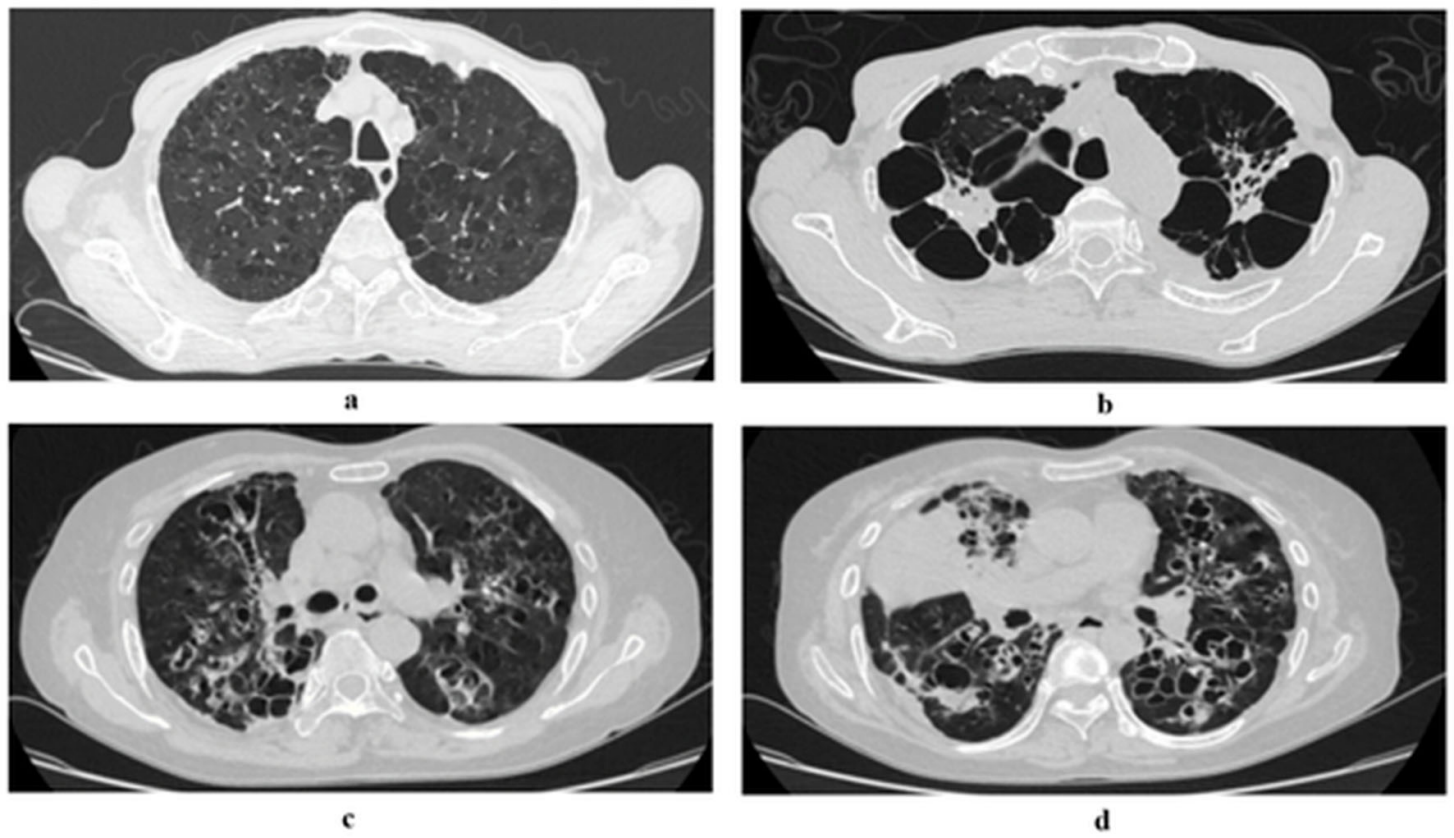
Examples of severity scoring for lung tissue damage. The HRCT scoring system in the provided CT images evaluates lung involvement extent (1–5 scale) and tissue damage severity (1–6 scale). Given that full CT scans are not available for all cases, extent of lung involvement scores are directly presented in the figure. The severity of tissue damage is categorized based on structural destruction and the percentage of lung area affected. (a) Mild emphysema and cystic lesions: The image shows mild emphysematous changes with minimal lung involvement. Severity of tissue damage score: 1. (b) Severe bronchiectasis with extensive cystic changes: The image demonstrates severe bronchiectasis with multiple cystic structures, significantly affecting both lungs. Severity of tissue damage score: 6. (c) Moderate bronchiectasis with widespread involvement: The image indicates moderate bronchiectasis with visible damage covering a substantial portion of the lung but not exceeding 80% of the total lung area. Severity of tissue damage score: 4. (d) Severe bronchiectasis with conglomerate masses: The image depicts severe bronchiectasis with complex cystic structures and masses, affecting over 80% of the total lung area. Severity of tissue damage score: 6.
